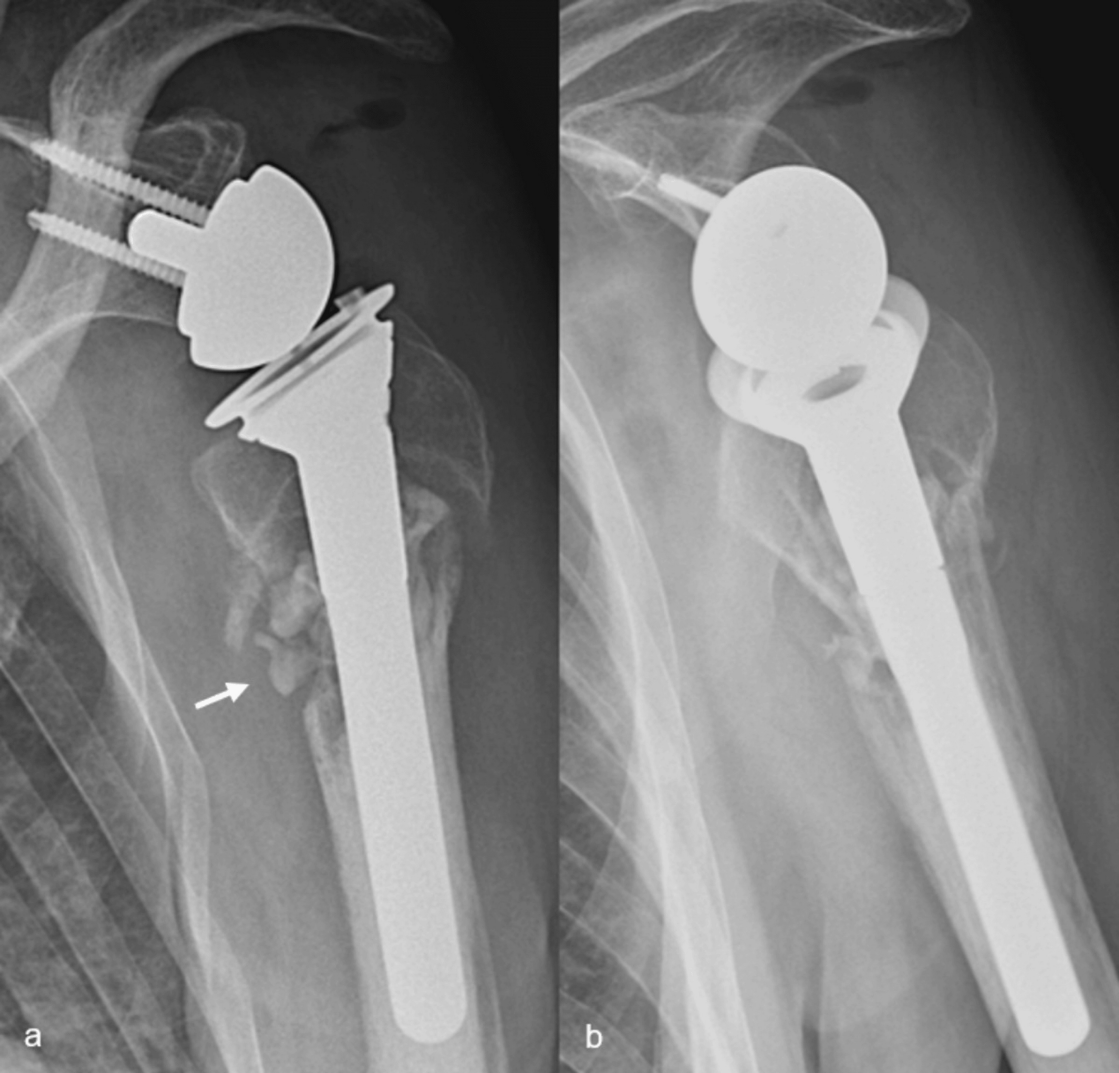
Shish kebab number two: the humeral stem is introduced through the proximal humeral fragment into the medullary canal Postoperative AP (a) and lateral (b) X-rays showing the humeral stem "shish kebabbed" through the proximal humeral fragment into the medullary canal. The distal part of the stem is cemented, while the fixation of the proximal part relies on its Trabecular Metal™ coating. There is some extrusion of cement into the non-union site (arrow).
